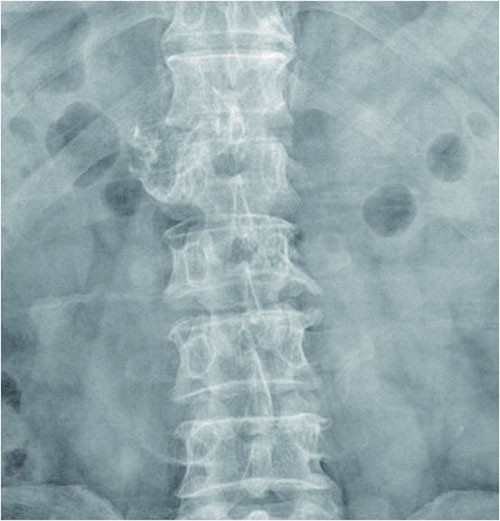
Anteroposterior thoracolumbar plain radiograph. The radiograph shows osseous exostoses on the right side of T12-L1.
